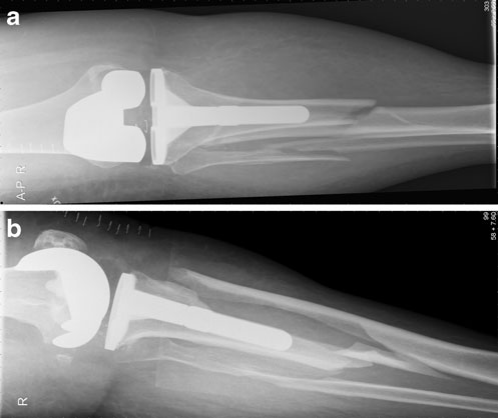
a, b Preoperative X-ray of a periprosthetic fracture around the tibial component of a TKA type Felix 3A; by Morten Schultz Larsen
